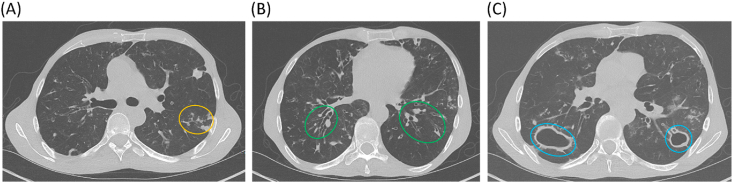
Imaging findings of autoimmune pneumonitis during first admission at the NIH. A. Radiographic features of APECED pneumonitis with ground glass opacities (GGO), tree-in-bud (TIB) opacities without bronchiectasis (yellow oval), and small nodular opacities. B. Bilateral bronchiectasis-associated structural lung disease and mucus plugging (green ovals). C. Large cavitary lesions caused by nontuberculous mycobacteria (NTM) (blue ovals). Chest CT performed in 2013. (For interpretation of the references to colour in this figure legend, the reader is referred to the Web version of this article.)
